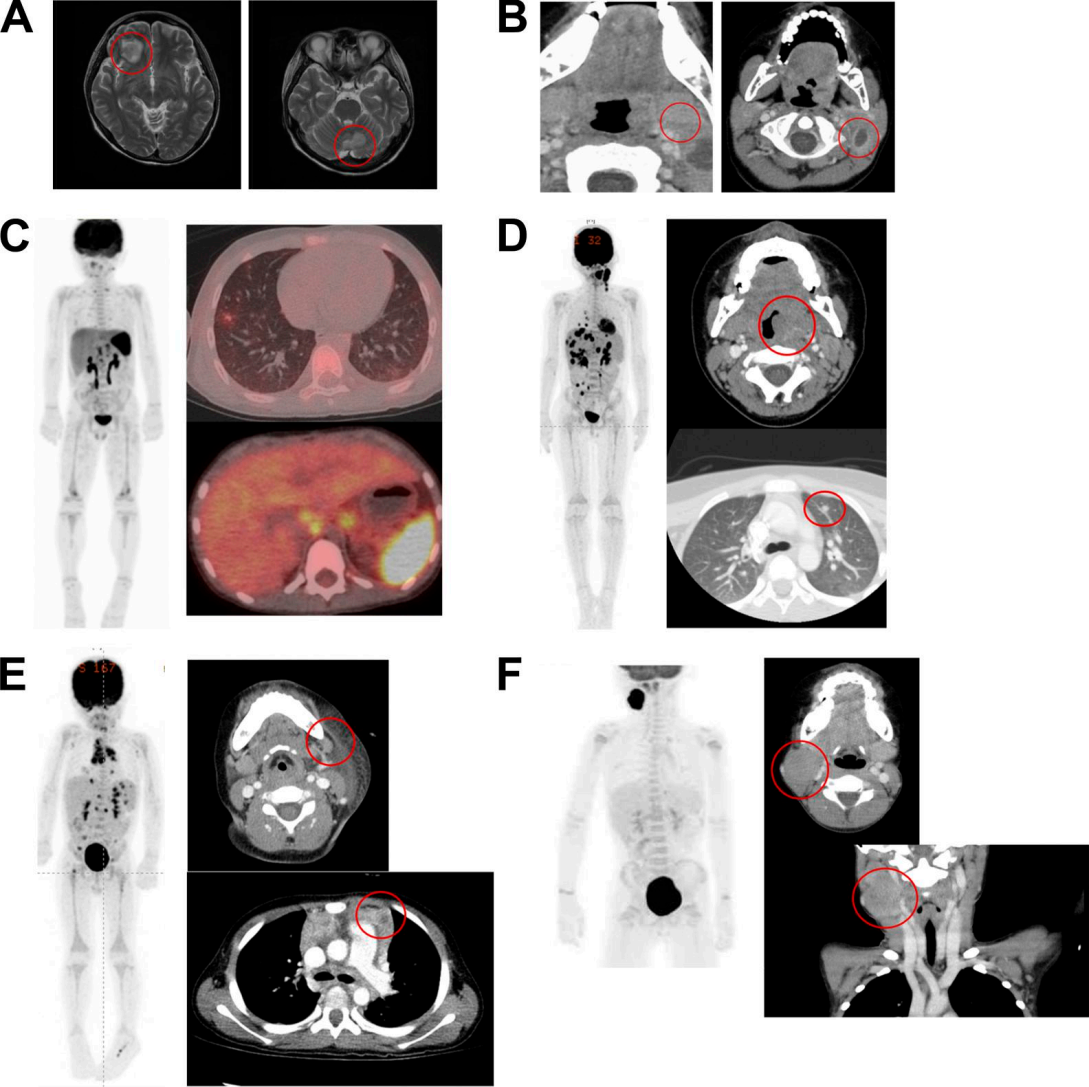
Imaging findings in patients with XLP1 presenting with LPD. (A–F) Magnetic resonance imaging T2-weighted images showing abnormal enhancement in the right frontal lobe (left panel) and cerebellum (right panel) in P1 (A). Contrast-enhanced computed tomography (CT) showing multiple enlarged left cervical lymph nodes in P2 (B). An FDG-PET image of the entire body in P3 is shown in the left panel. PET-CT images show high enhancement in the right lung (upper right panel), para-aorta, and liver, with extremely high enhancement in the spleen (lower right panel) (C). An FDG-PET image of the entire body of P4 is shown in the left panel. Contrast-enhanced CT images show enlargement of the left tonsil (upper right panel) and a nodule in the left lung (lower right panel) in P4 (D). An FDG-PET image of the entire body of P5 is shown in the left panel. Contrast-enhanced CT images show enlargement of the left cervical lymph node (upper right panel) and para-aortic lymph node (lower right panel) in P5 (E). An FDG-PET image of the entire body in P6 is shown in the left panel. Contrast-enhanced CT showing enlarged right cervical lymph nodes (upper and lower right panels) (F).
